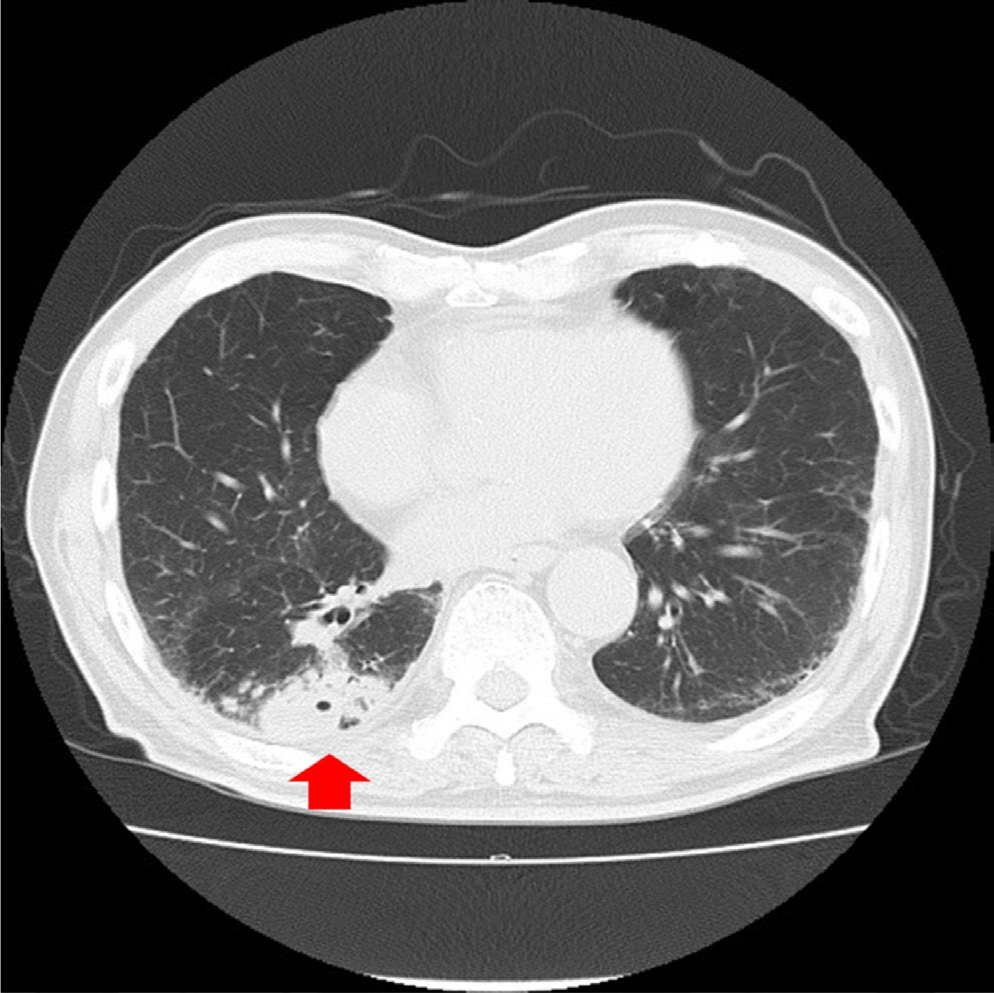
Chest computed tomography (CT) scan of an 85‐y‐old male with intraductal papillary mucinous neoplasms (IPMN) of the pancreas showing a mass with a cavity and surrounding nodules in the right lower lobe, which was diagnosed as *Mycobacterium tuberculosis*
